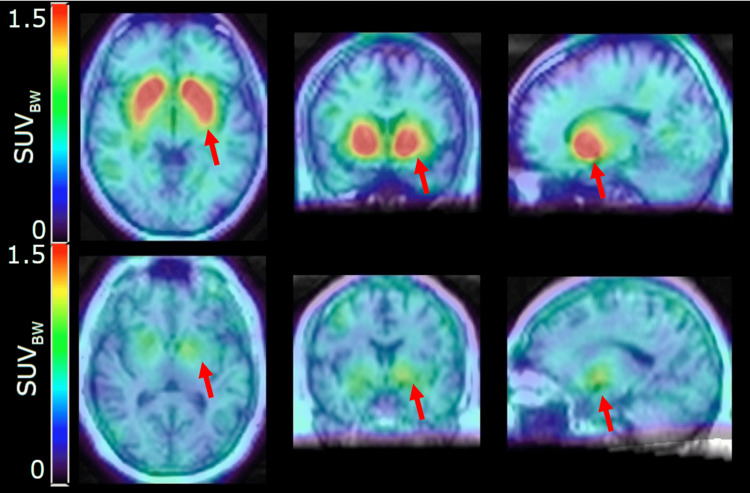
The SUVBW images overlay on the MRI of one of the normal subjects (top row) versus one of the kratom-dependent subjects (bottom row) for axial, coronal, and sagittal slices. Kratom-dependent subjects consumed the kratom for 21 years of duration. Images were reconstructed using 4i10s (Butterworth filter frequency of 0.4 and an order of 10) for image display. Red arrows represent the striatum region for normal subjects and kratom-dependent subjects, respectively. SUV_BW_: standardized uptake value of body weight
